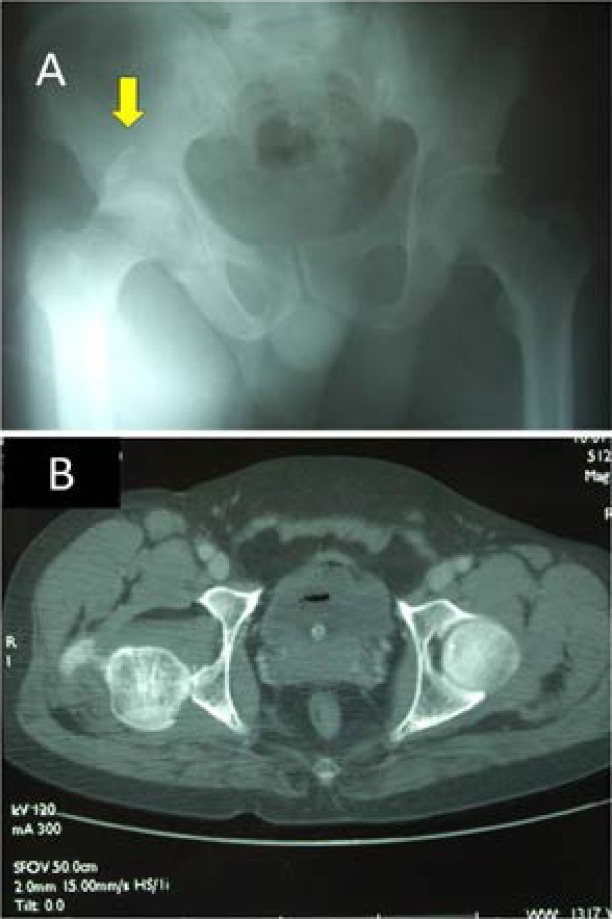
30-year-old male front-seat passenger was involved in a frontal impact road traffic collision. He sustained a posterior dislocation of the right hip, fracture of the posterior rim of the right acetabulum (yellow arrow) (A, B) and a right intra-capsular neck of femur fracture. Notice the lateral orientation of the acetabulum which is shown in the CT scan (B).
